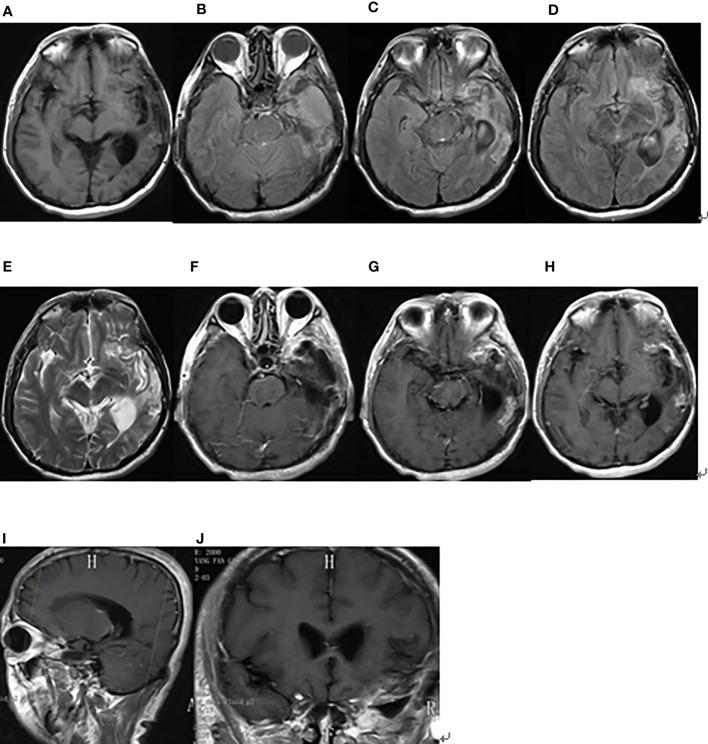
Brain MRI findings after the second surgery on December 4, 2019. (A) The axial plane of T1-weighted MRI. (B–D) The axial planes of T2-weighted FLAIR MRI. (E) The axial plane of T2-weighted MRI. (F–H) The axial planes of enhanced T1-weighted MRI. (I, J) The sagittal and coronal planes of enhanced T1-weighted MRI.
